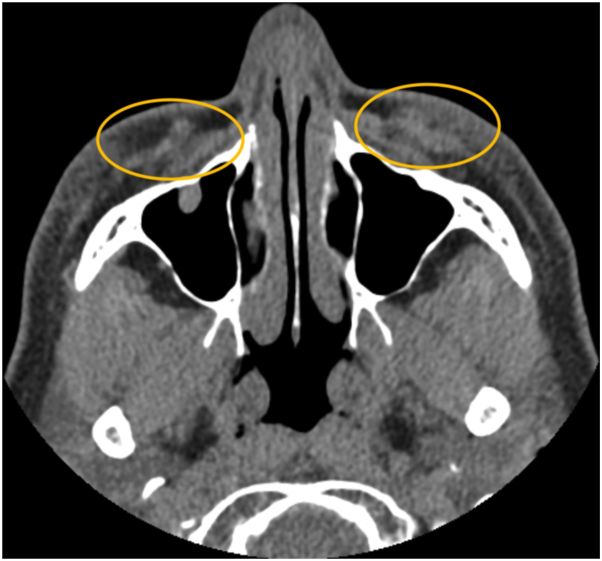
Masses in the bilateral tear troughs on computed tomography scan. Sample image from the patient’s computed tomography scan. Masses (*circled*) are integrated into the skin in the regions injected with hyaluronic acid filler.
